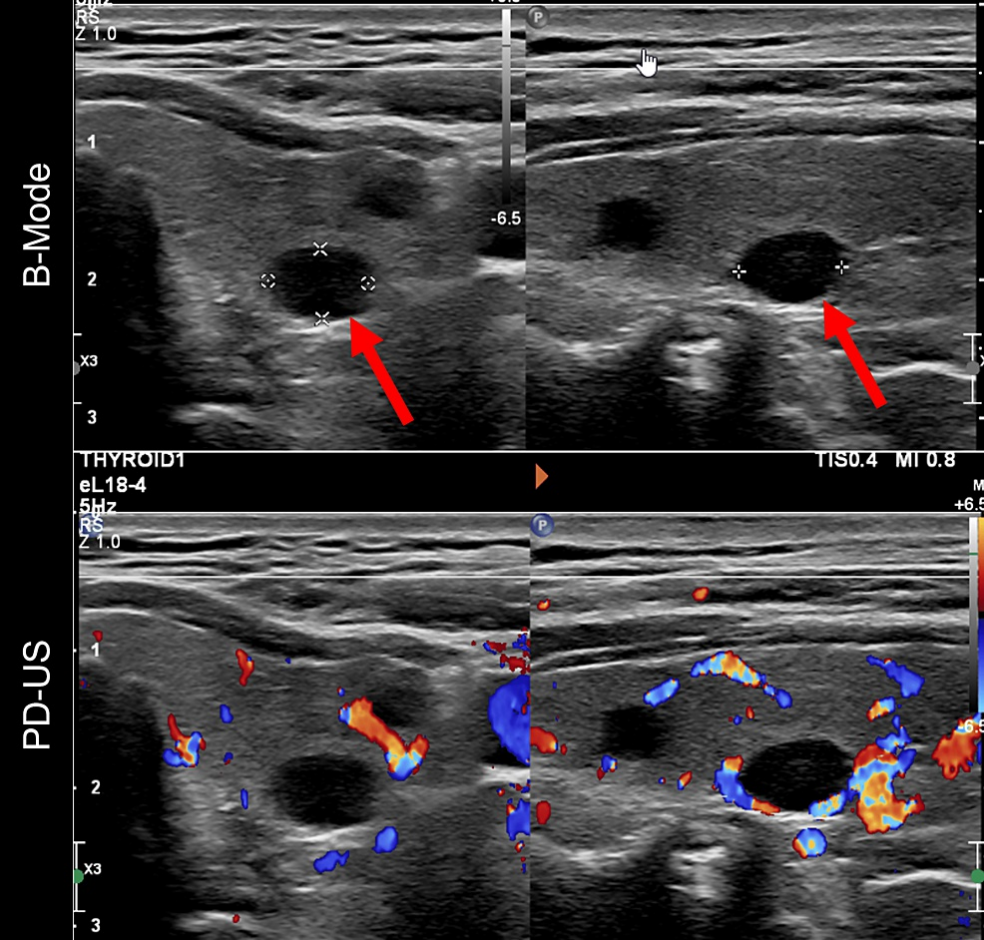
Ultrasound of the thyroid showing a 0.8 cm hypervascular solid nodule in the left lower pole
